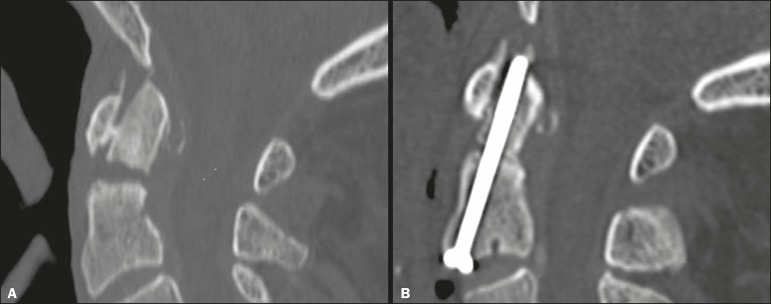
Head CT including the C1-C2 region in an 80-year-old patient with frontal
